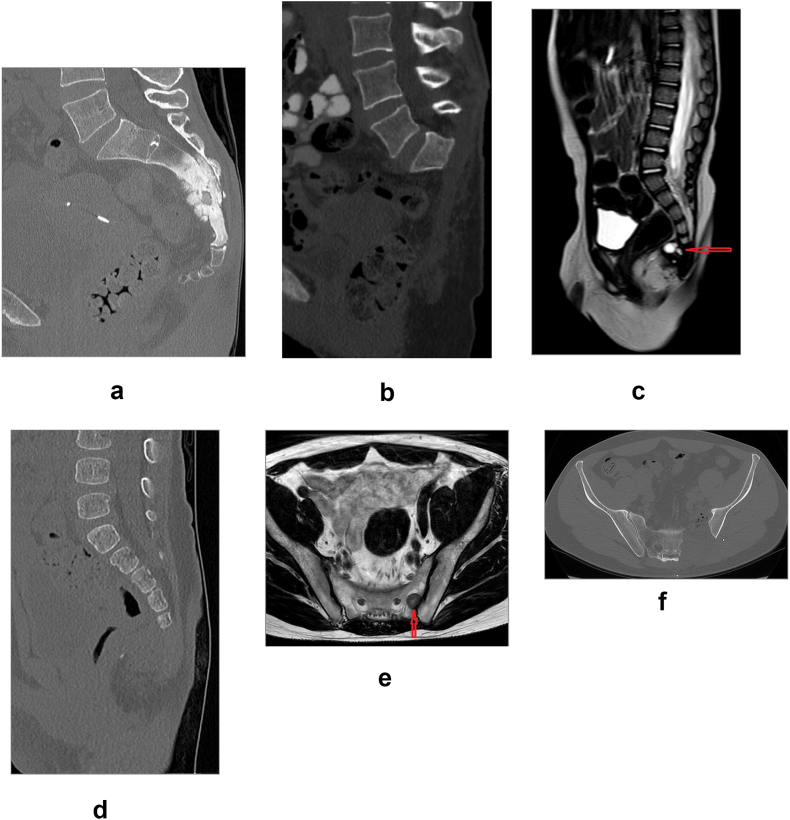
Examples of different levels of resection. CT reconstructions in sagittal plane of high level resection (S1-S2) for osteosarcoma in 51 + 7 female patient pre- (a) and postoperatively (b). 1 + 4 male patient with yolk sac tumor (red arrow) preoperative MRI T2-weighed sagittal image (c) and CT reconstruction in sagittal plane after low level resection (S4-S5) (d). 48 + 3 male patient with leiomyosarcoma metastasis (red arrow) in T2-weighed axial cut of MRI (e) after unilateral resection in axial cut of CT reconstruction (f).
